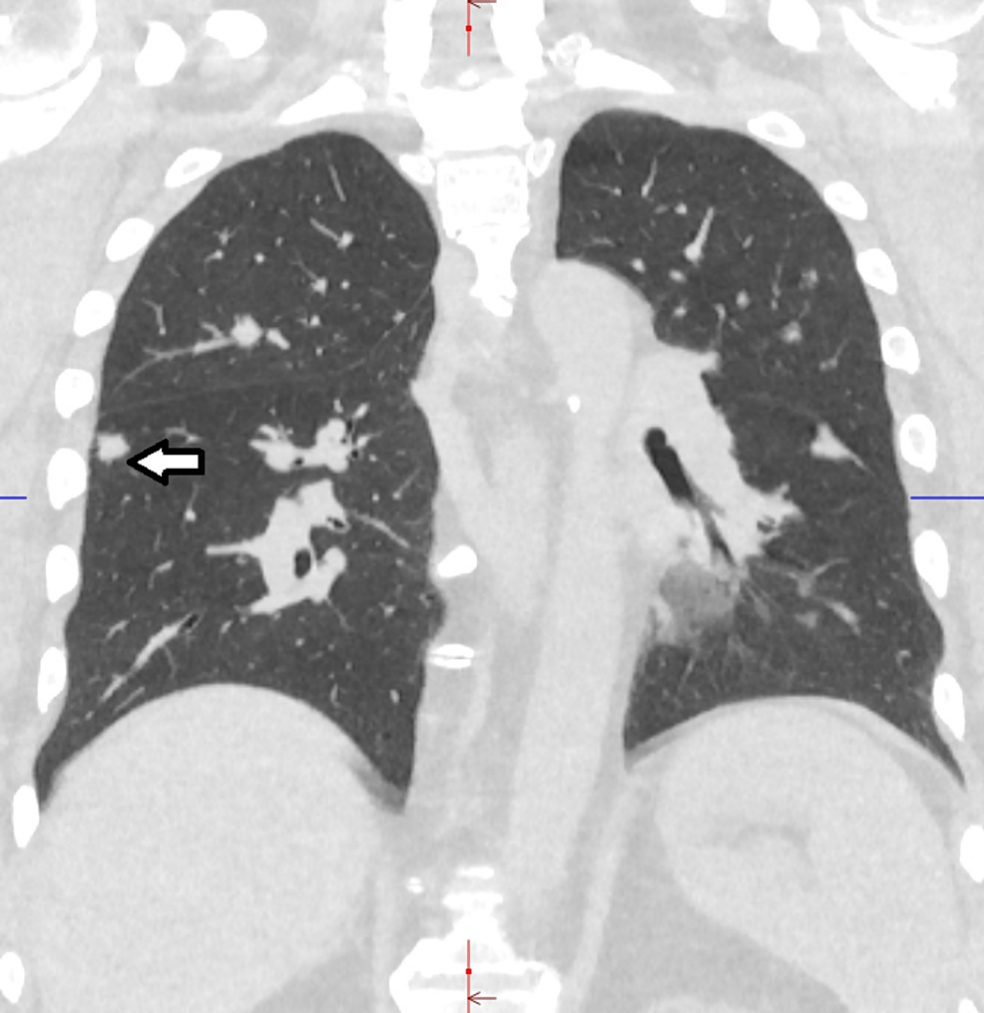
CT chest (lung window) (coronal view). Right lower lobe nodule (arrow).
